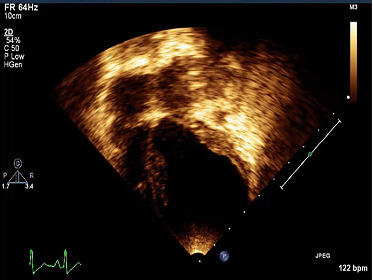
Doppler echocardiography reveals a severe DCM in the proband. Four chambers' view of the echocardiography allows to appreciate the left auricle and the left ventricle severely enlarged and the thinned interventricular septum bulging into the right ventricle.
